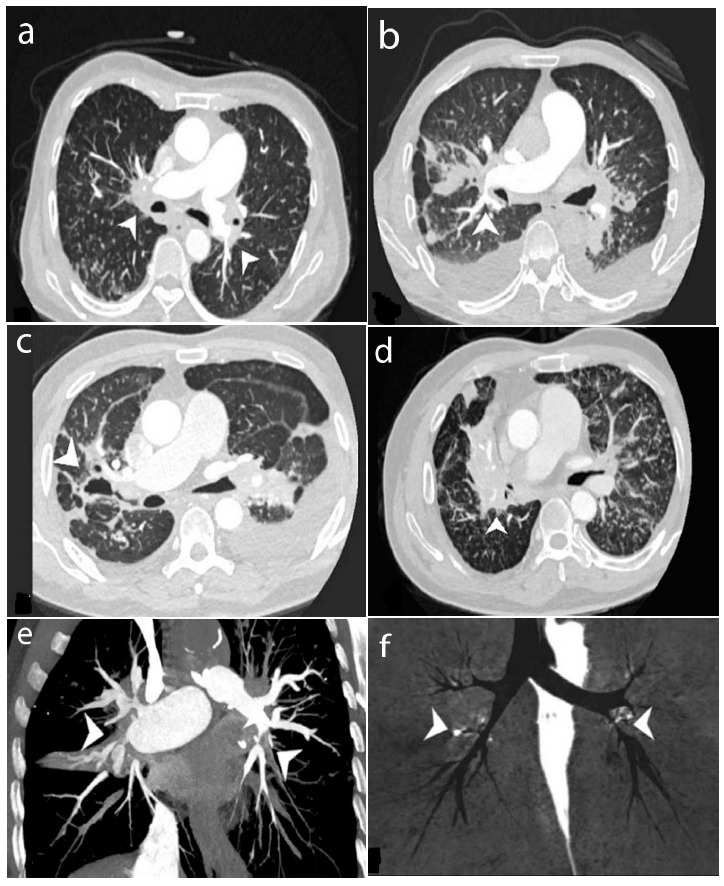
HRCT of TB-FM (different patients). (a-d) Fibrosis tissue is located at both hilar, causing stenosis of the pulmonary arteries and bronchus (marked by a white arrow). Consolidation (b-c), cavitation (c), pleural effusion (b-c), and Centrilobular nodules (d) are also shown (white arrow). (e, f) Bronchial and pulmonary arteries in the same segment are prone to stenosis (displayed by a white arrowhead).
